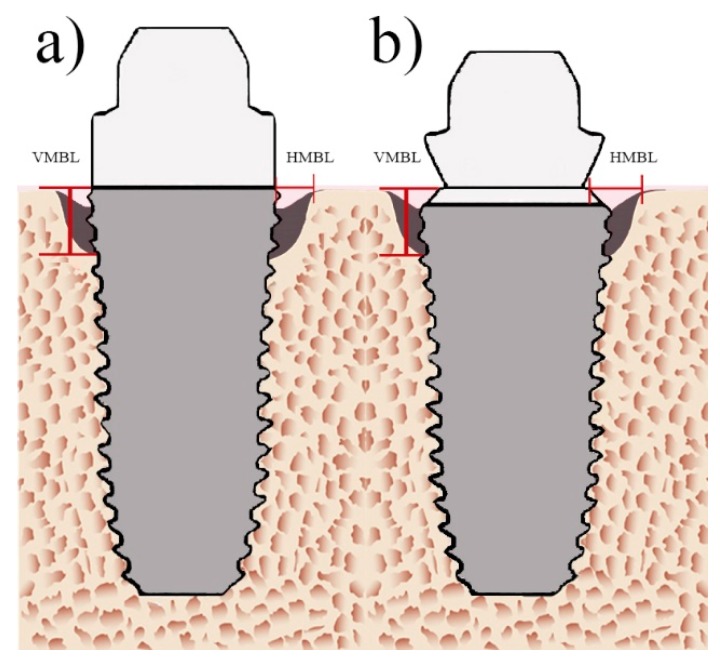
Radiographic measurements of platform-switched implants. (a) Platform-matched (PM) dental implants, (b) platform-switched (PS) dental implants. Vertical marginal bone loss, VMBL; horizontal marginal bone loss, HMBL.
